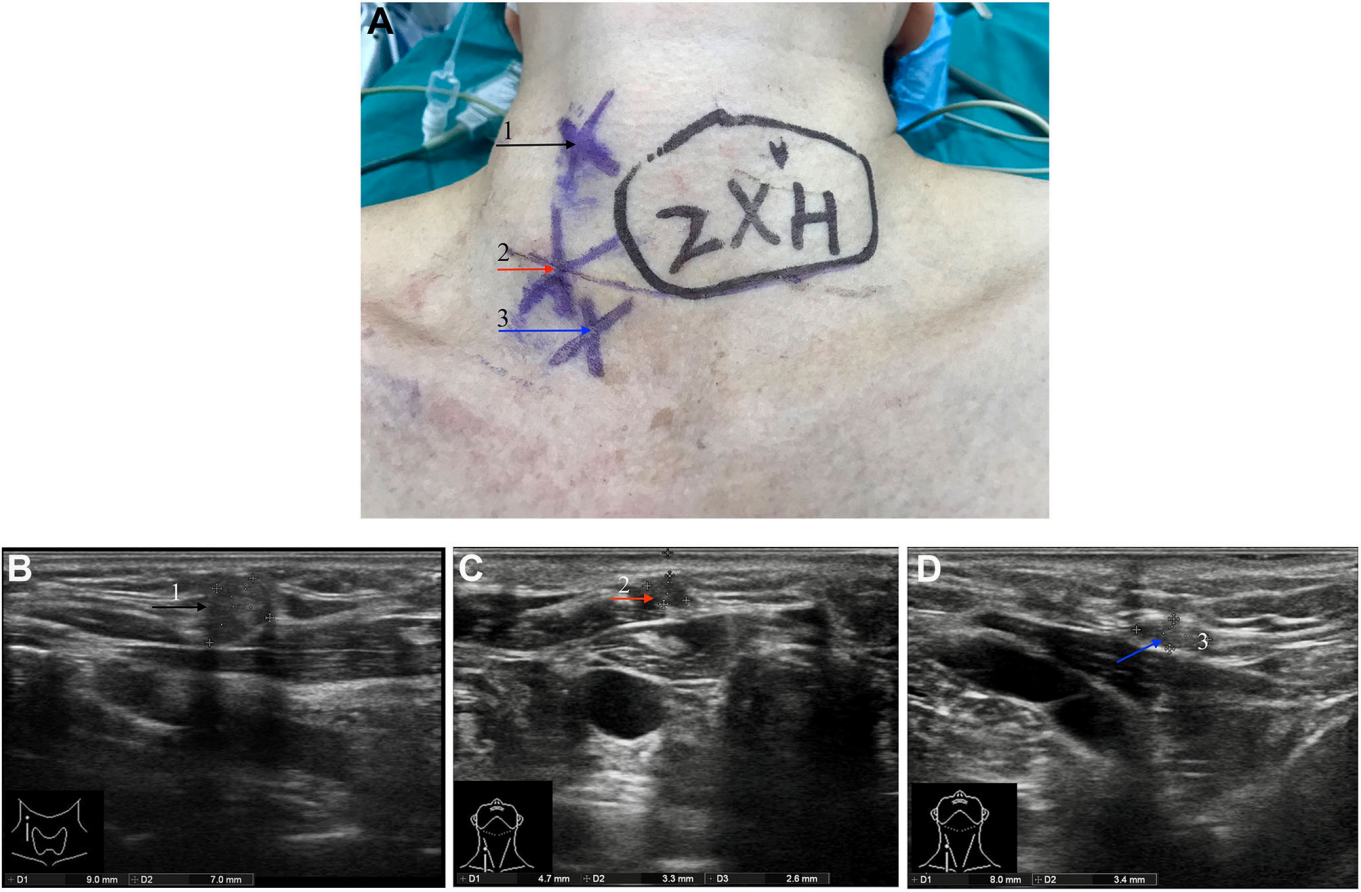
Pre-operative physical examination and ultrasound examination. (A) Three subcutaneous palpable nodules at the right anterior neck and the right supraclavicular. The neck ultrasound showed two hypoechoic nodules with a size of about 9^*^7^*^9 mm (F) and 5^*^3^*^7 mm (G) subcutaneously at the right anterior neck. A hypoechoic nodule with a size of about 8^*^4^*^6 mm (H) was observed at the right supraclavicular. Three nodules are annotated with black, red, and blue arrows, respectively, and corresponding the nodules indicated by the arrow in (A).
